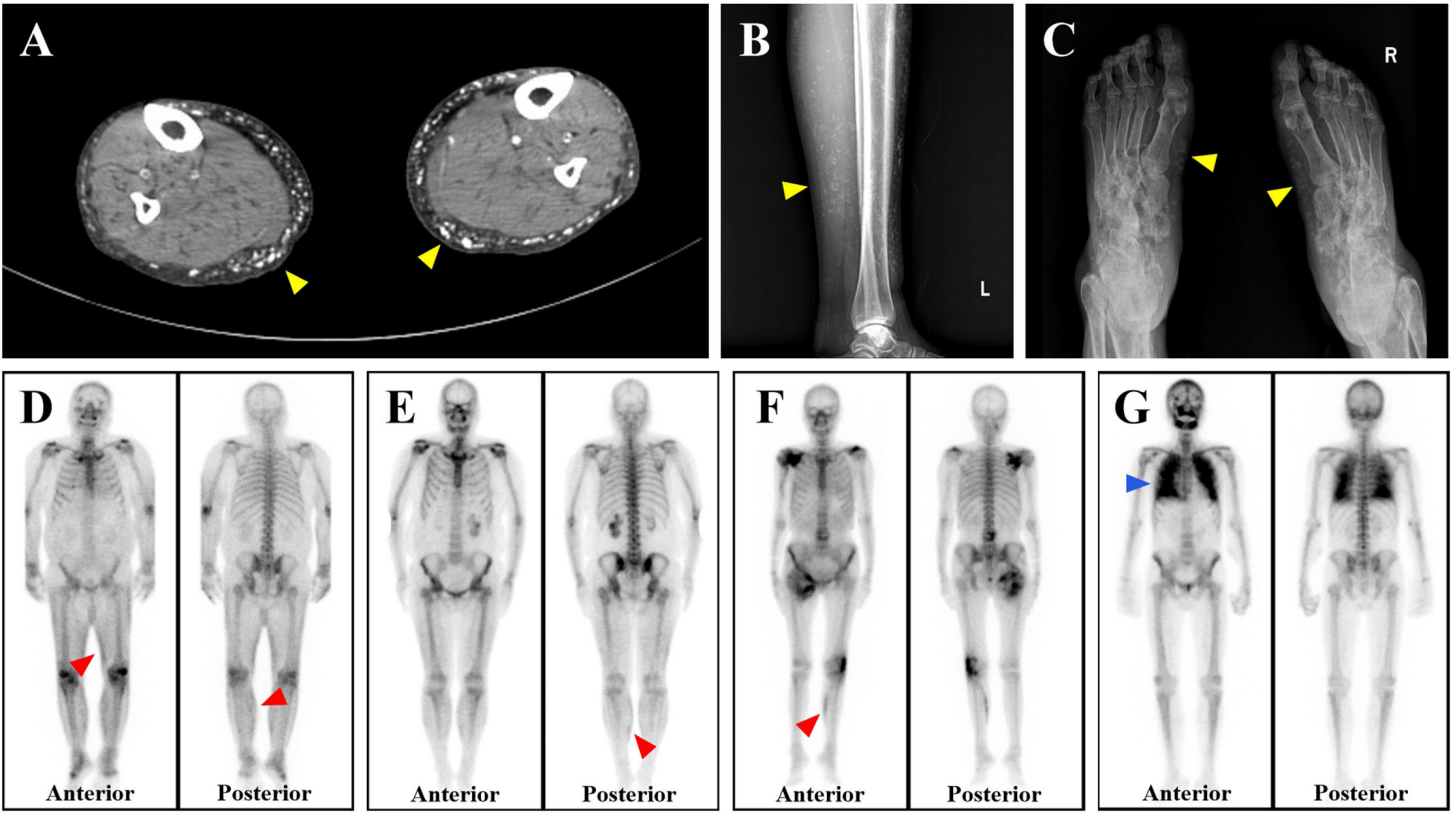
Imaging characteristics of calciphylaxis. (A–C) Both CT and X-ray reveal subcutaneous extravascular diffuse calcium deposition of patients with calciphylaxis (yellow arrows, shown as white). (D–F) Bone scintigraphy shows the increased uptake of radiotracer by subcutaneous soft tissues of three calciphylaxis patients, especially the continuous linear abnormal radioactive concentration in the lower limbs (red arrows, shown as black). (G) Diffuse uptake enhancement is also observed with bone scintigraphy when calciphylaxis involves internal organs such as the lungs (blue arrows).
